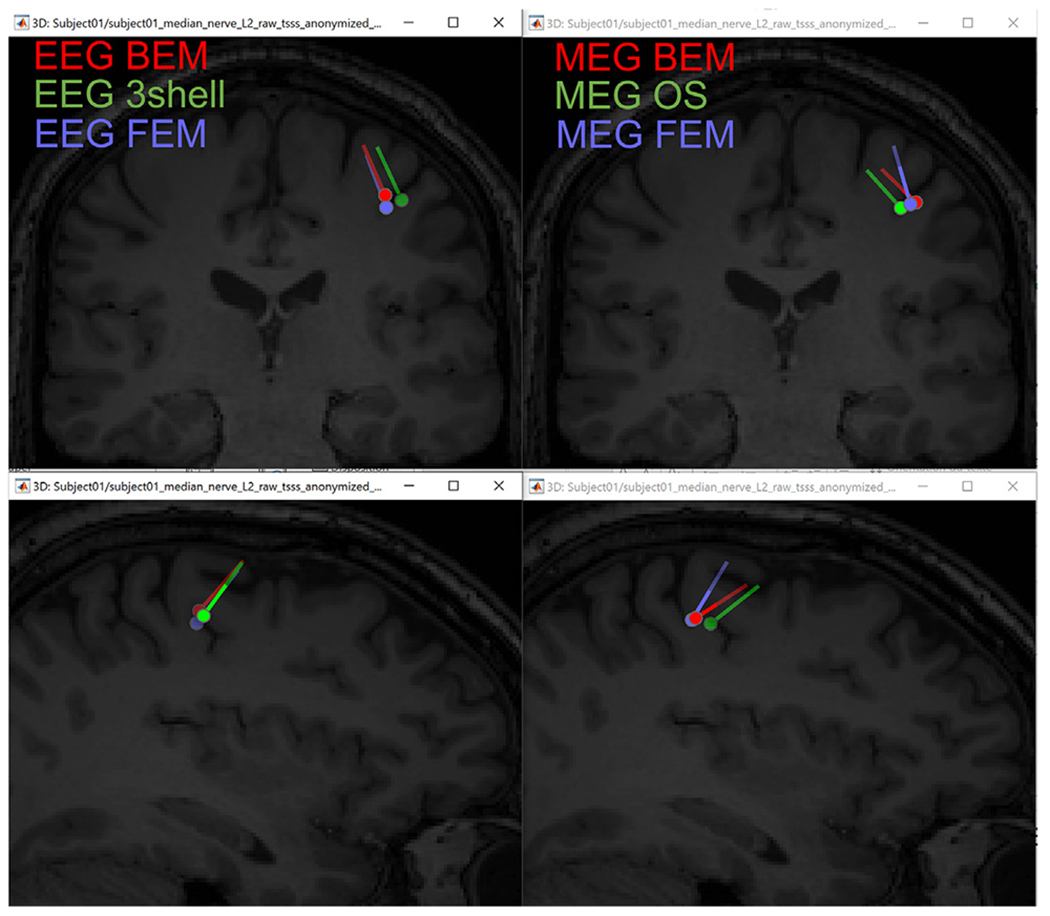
Reconstructed source of the P20/N20 component and its location within the source space displayed on the MRI (top: coronal; lower: sagittal). Left are the EEG-based dipoles. Right, are the dipoles from MEG. Green dipoles were computed from the analytical methods (overlapping spheres for MEG and 3shell for EEG), red from the BEM, and purple from the FEM.
